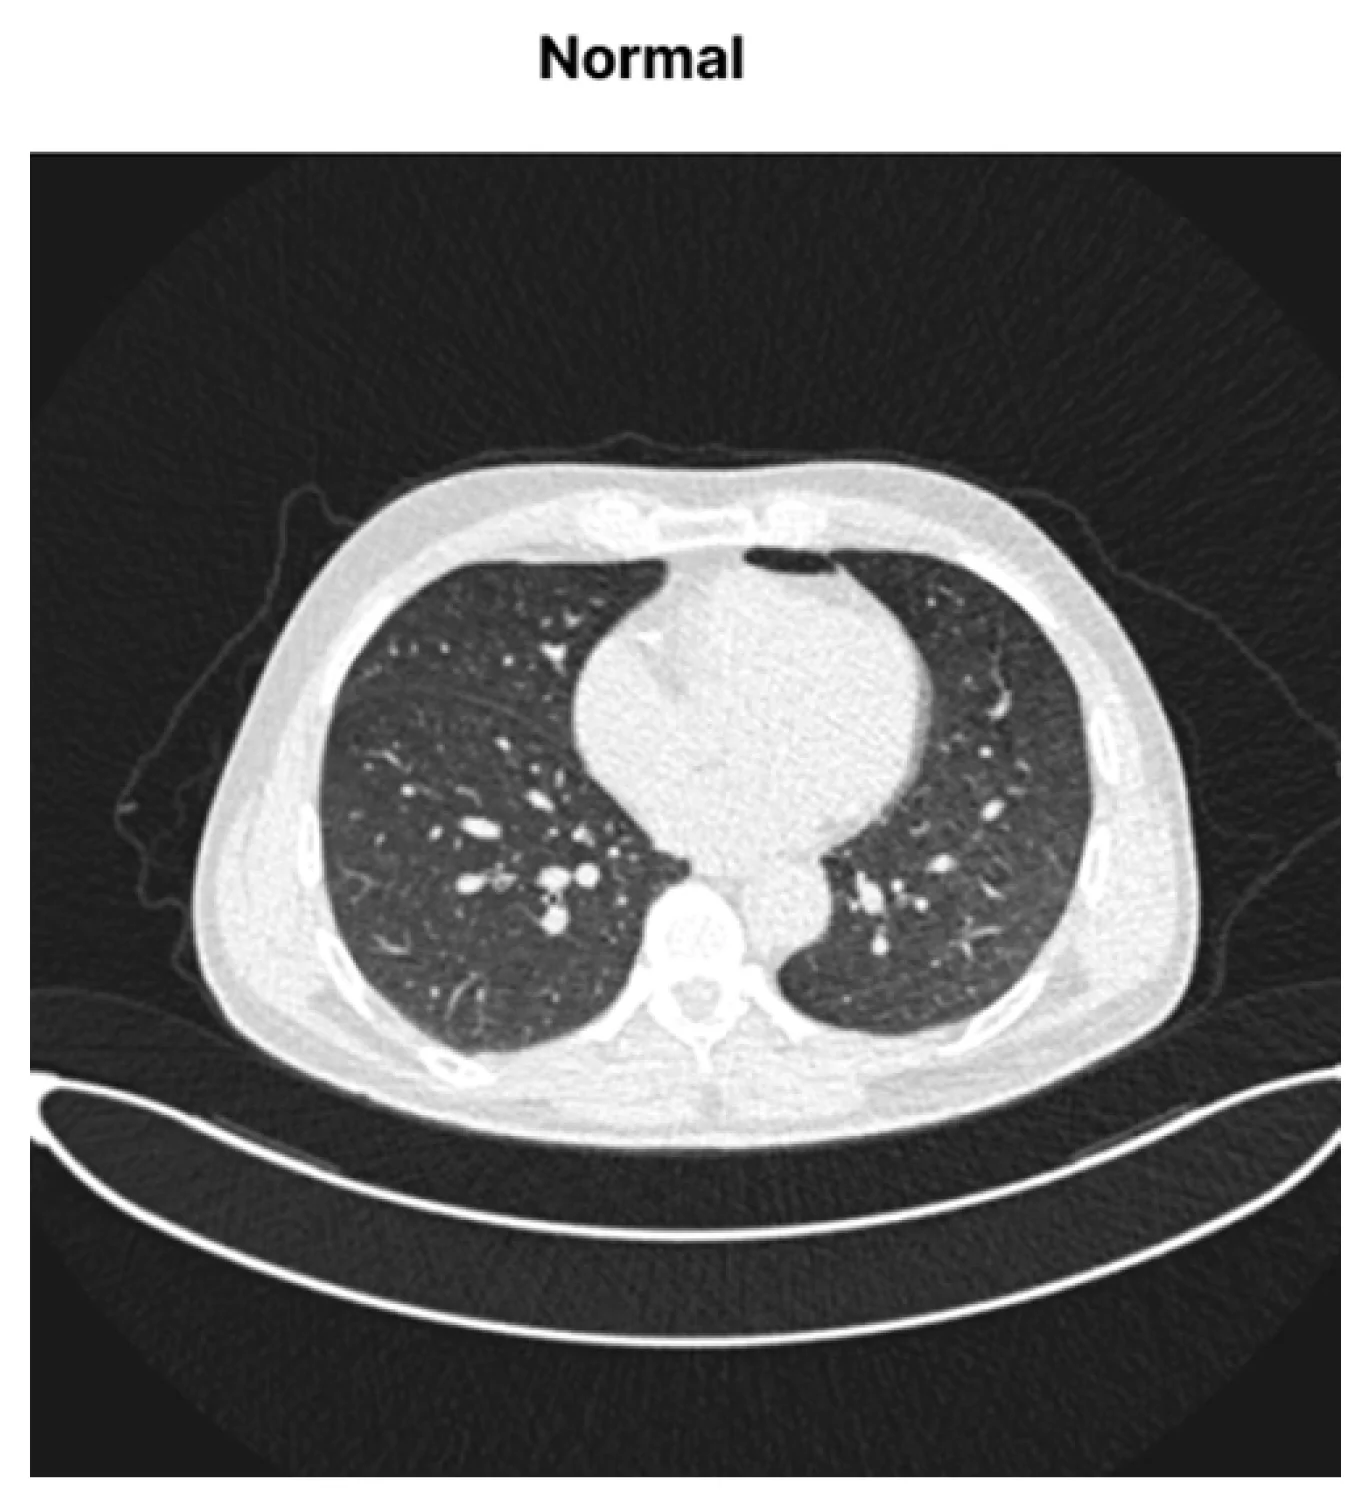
Sample CT scan of normal lung tissue.
